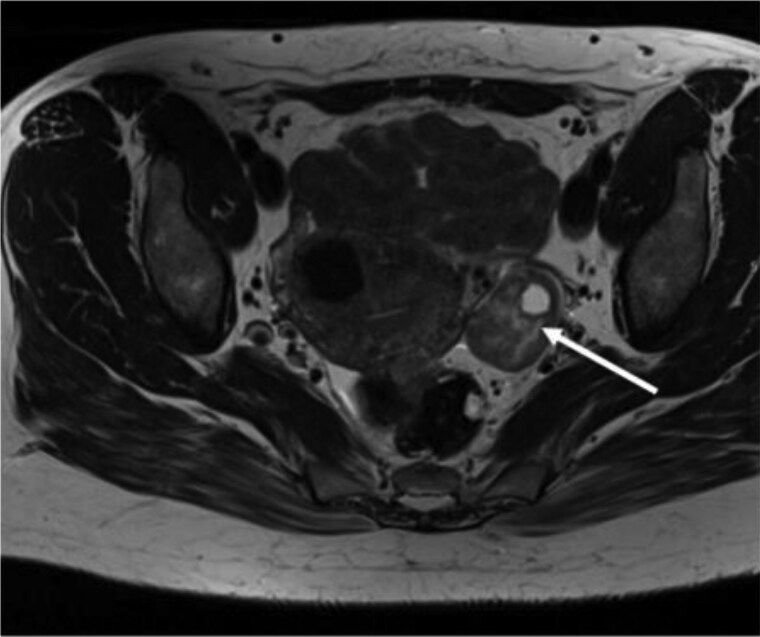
T1-weighted magnetic resonance imaging of the pelvis demonstrating a left adnexal mass in Case 2. The arrow points to the mass.
